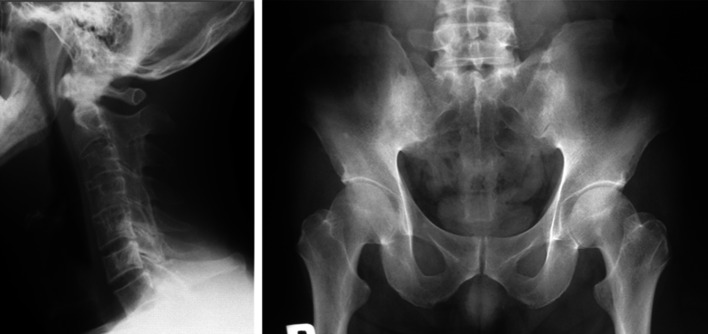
Radiography of the bamboo cervical spine (*left*) and bilateral sacroiliac joints (*right*) of case 1
